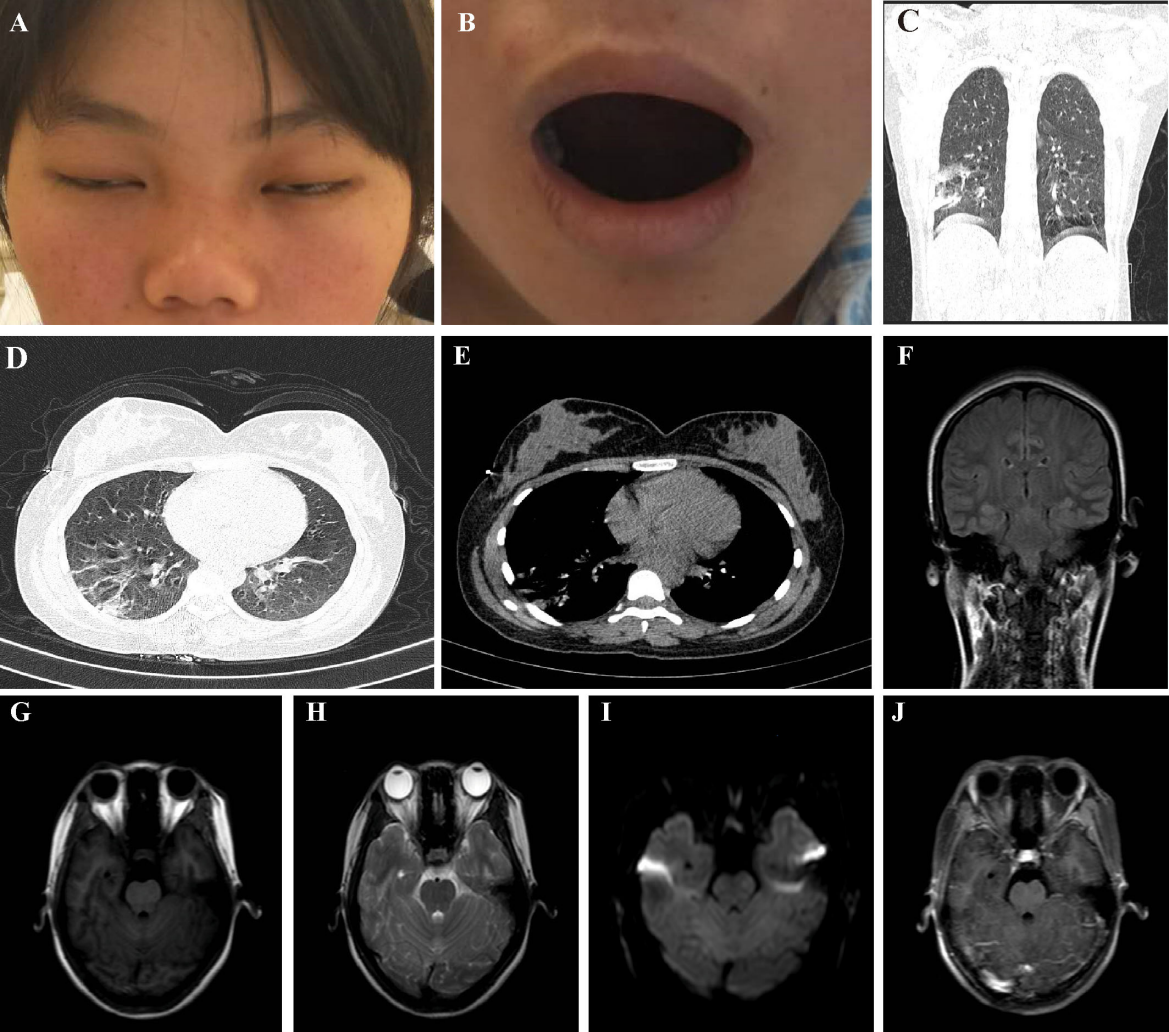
Clinical examination reveals dysfunction of facial nerve and the motor branch of the trigeminal nerve. (A) Bilateral facial weakness and Bell’s sign, (B) Limited mouth opening (tried the most effort to open her mouth); (C-E). Multiple lesions in the lower lobe of the right lung; (F-J). Head magnetic resonance imaging was normal.
